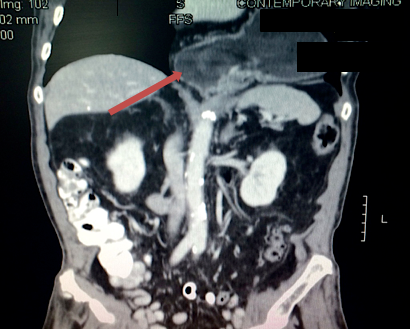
CT Scan of Abdomen Showing the Stomach Completely Above the Diaphragm.
